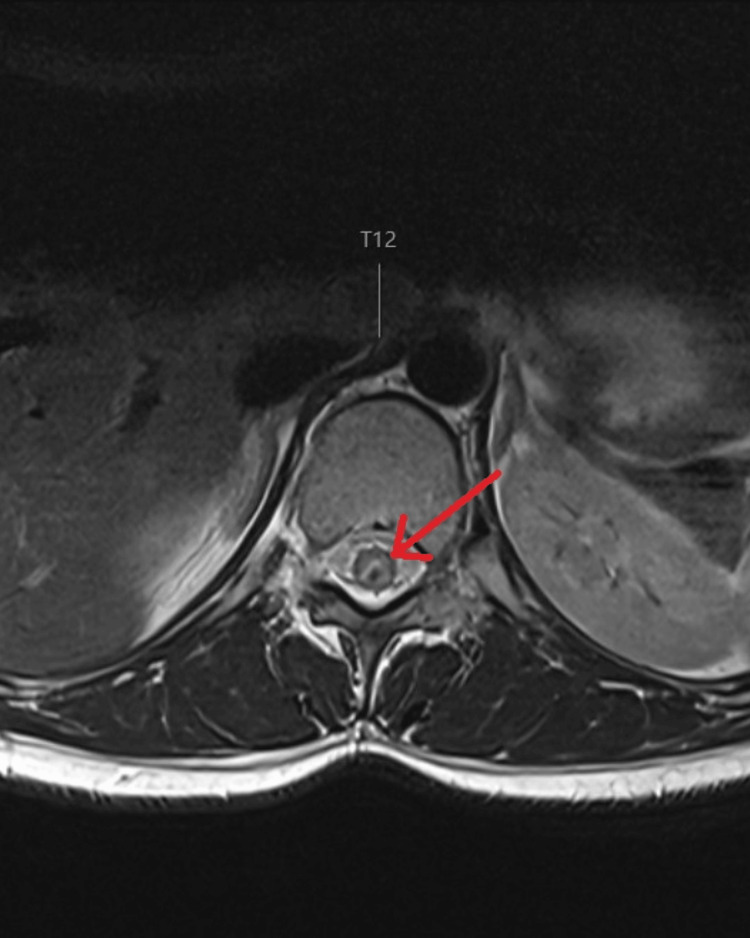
T2-weighted MRI showing axial section of the thoracic spinal cord at T12 level, with dorsally located, isointense lesion with a rim of hyperintense CSF bordering the lesion Red arrow mark showing the intramedullary spinal cord lesion at the level of T12 vertebra
